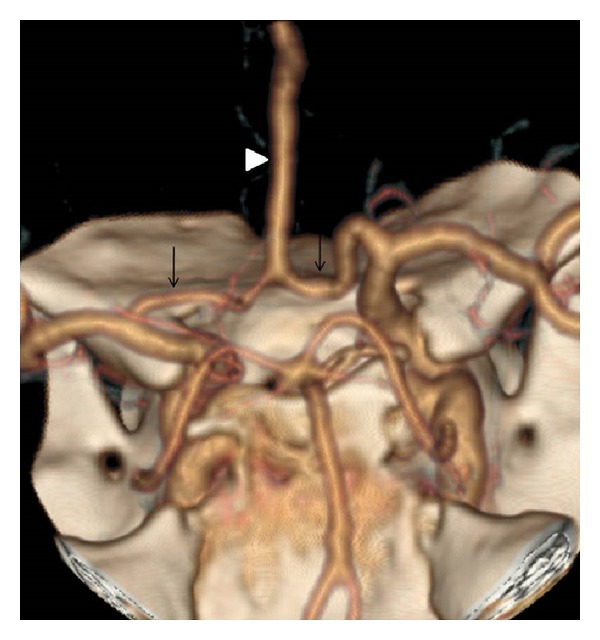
Computed tomographic angiogram showing the azygous A2.
